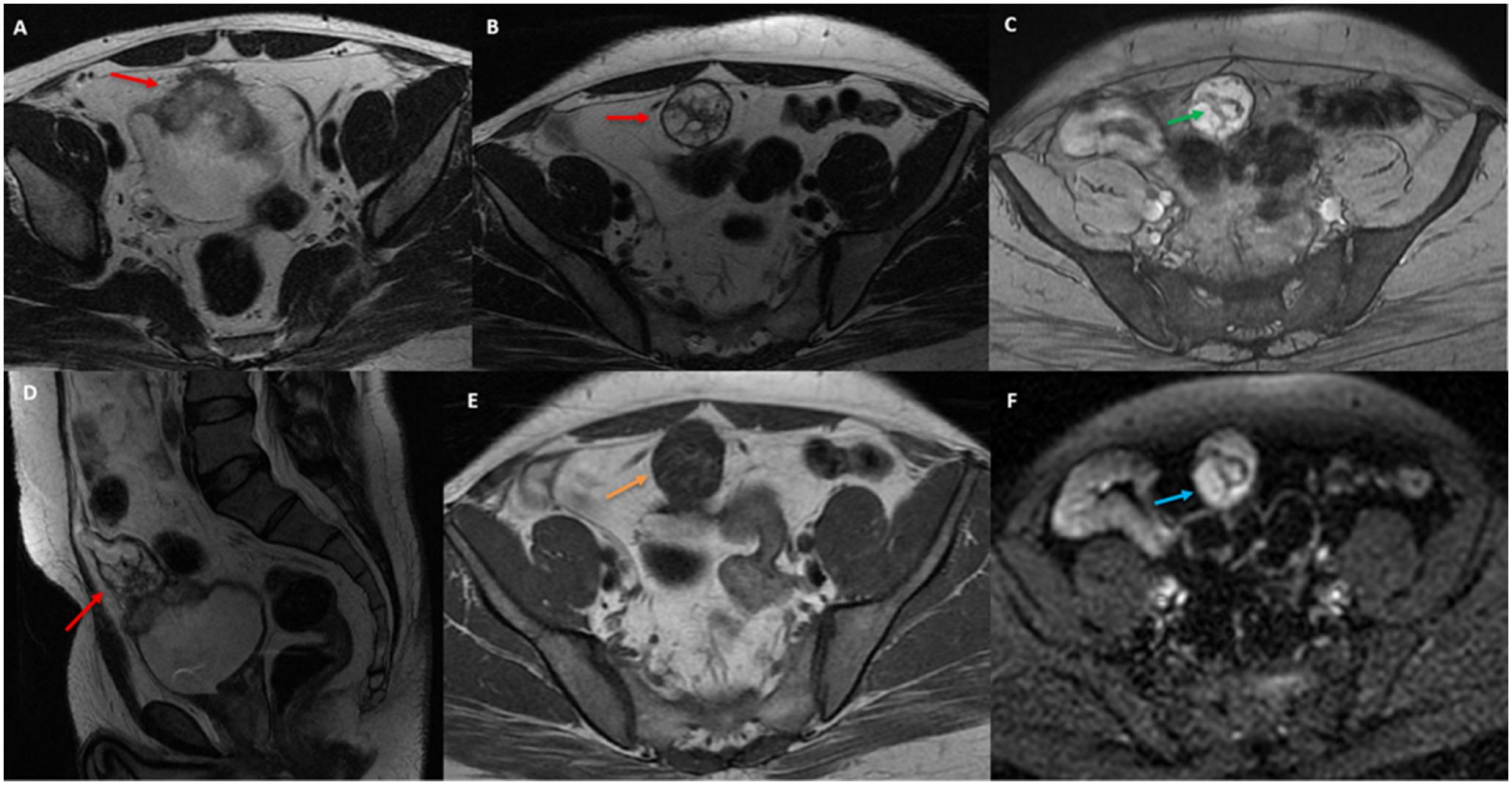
On MRI, (A, B) axial T2W, (D) Sagittal T2W sequences show a heterogeneously hyperintense mucinous lesion (red arrows) with hypointense wall, septations, and calcifications. (E) On axial T1W, the lesion appears heterogeneously hypointense (orange arrow). (C) GRE sequence shows blooming from calcifications (green arrow). DWI reveals restricted diffusion (blue arrow) indicating high cellularity, malignancy.
